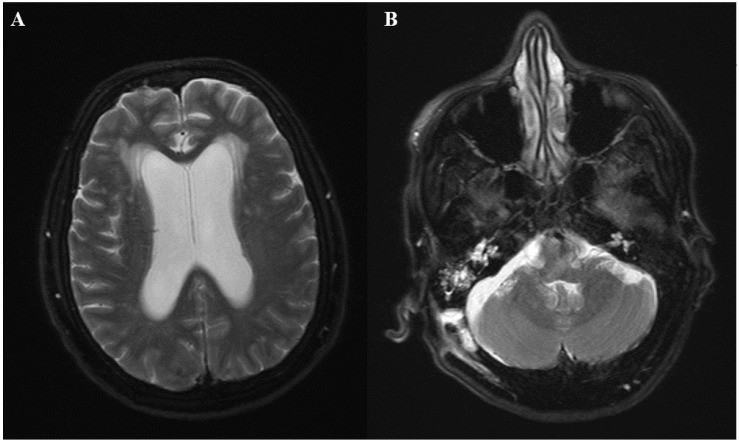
(A) Axial T2-weighted MRI (magnetic resonance imaging)showing diffuse leptomeningeal enhancement and moderately dilated ventricles (B) T2-weighted MRI showing a right cerebellar extra-axial collection of approximately 1 cm in diameter.
